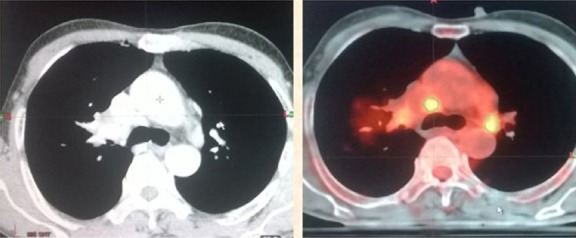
GTV lymph node volume drawn in red on CECT and green on ^18^F-FDG PET-CT showing increase in GTV volume on^18^F-FDG PET-CT
